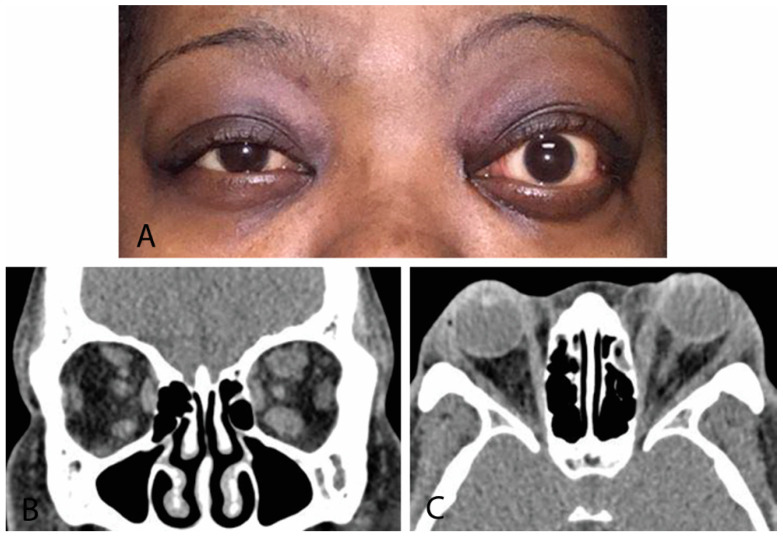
(A) External color photograph of chronic moderate thyroid eye disease with asymmetric proptosis on left more than right. (B) Coronal CT of orbits with thickening of recti muscles on left more than right. (C) Sagittal cut of same CT scan.
